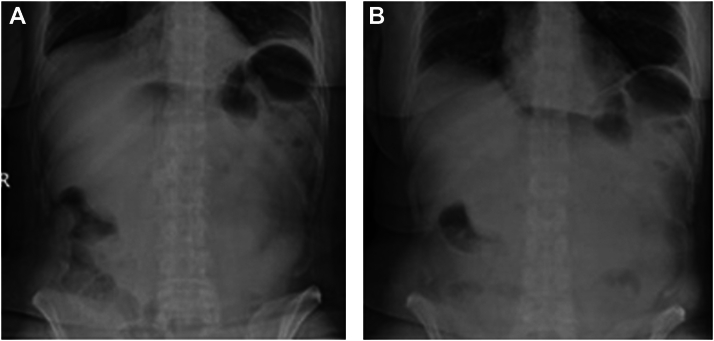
(A) First normal abdominal X-ray before EGD. (B) Second normal abdominal X-ray before EGD.
